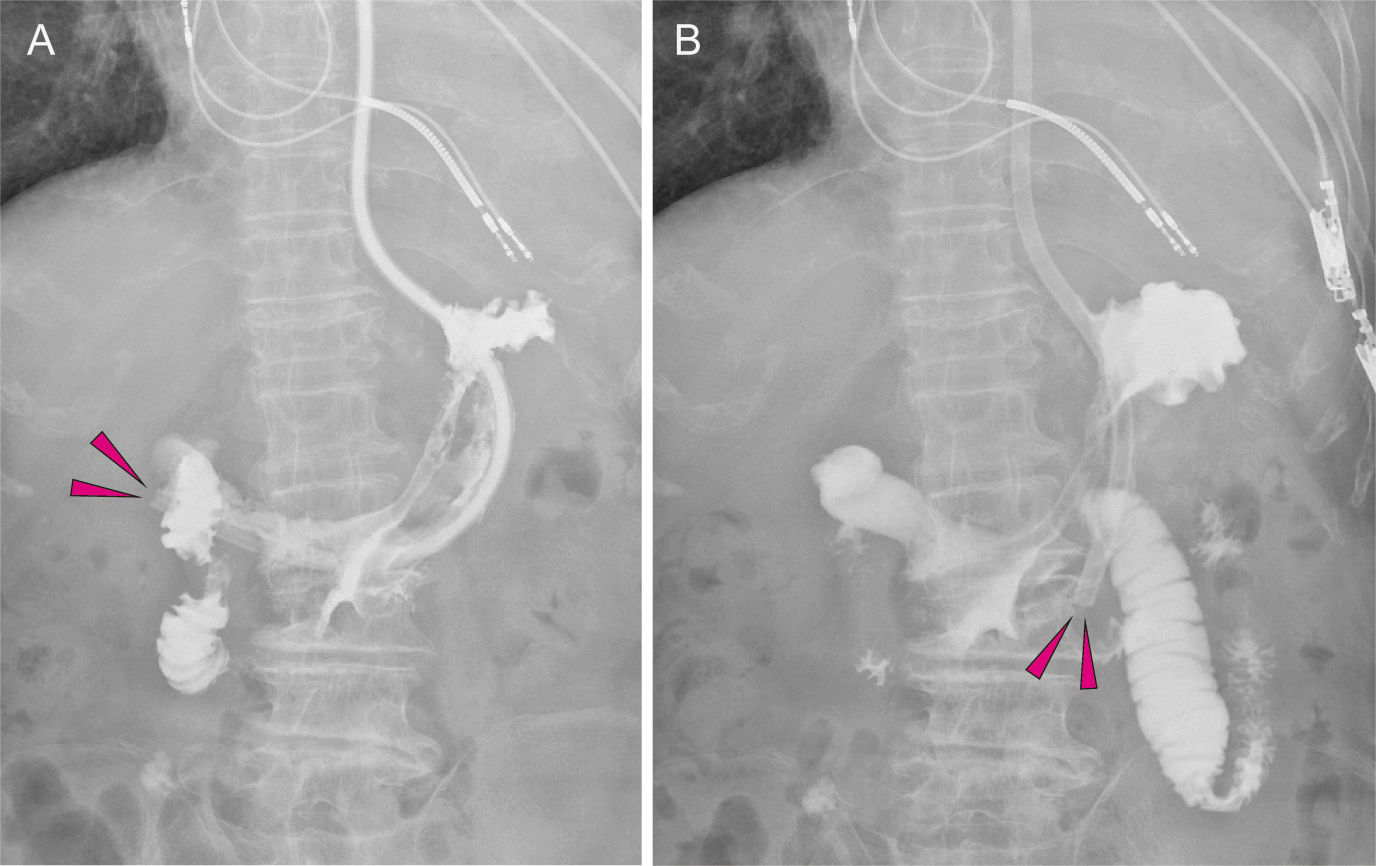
A: Gastrointestinal radiography using a nasogastric tube. The nasogastric tube tip (arrows) is positioned at the gastric pylorus, whereas the duodenum is immediately in contrast. The tube tip position progressed from the initial position, presumably via peristalsis. B: The nasogastric tube tip (arrows) was pulled back into the gastric body. The tube was adjusted and fixed in the nasal cavity at 55 cm.
